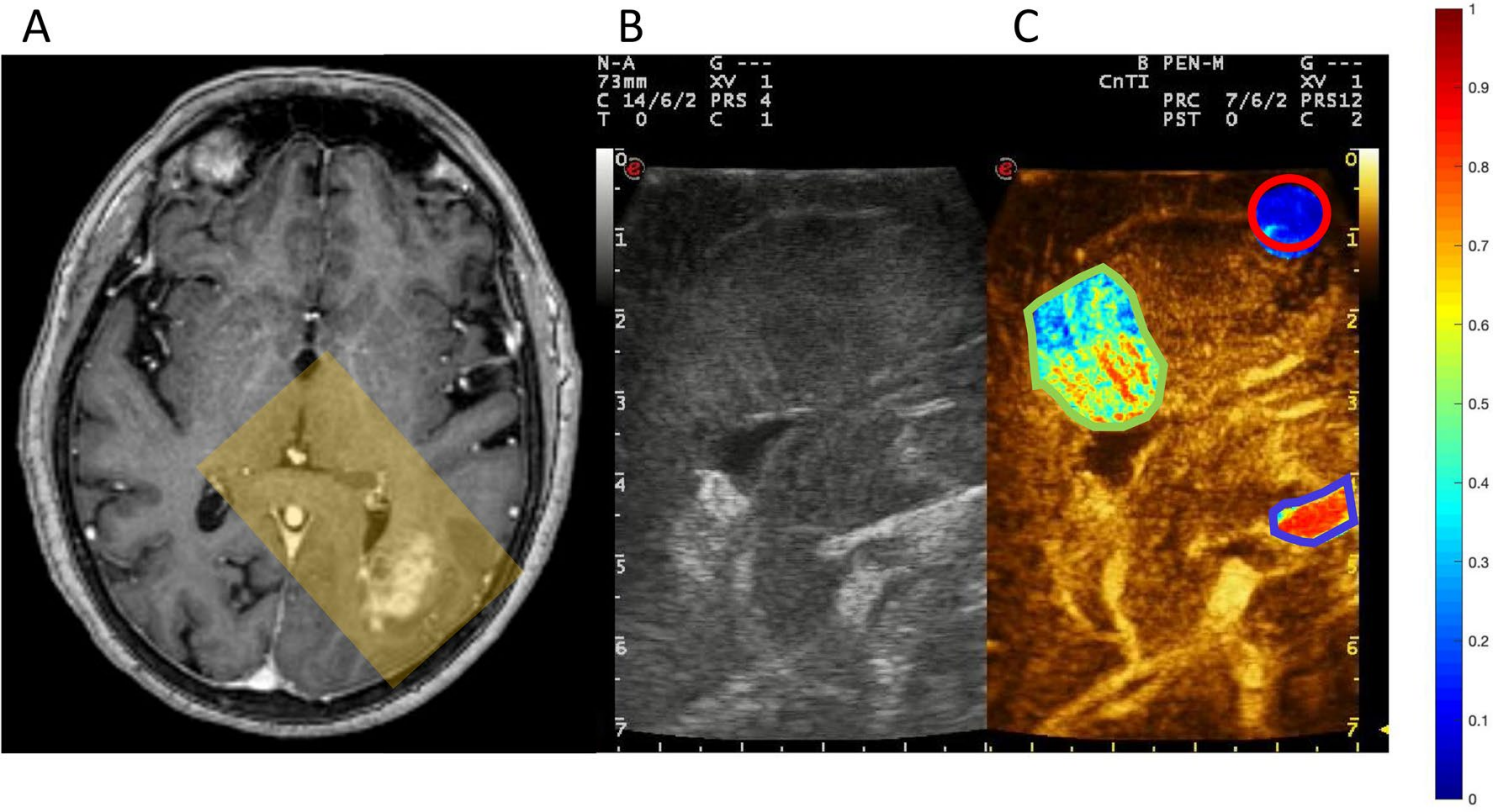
Qualitative view of microbubble density in selected regions in a case of parietal glioblastoma: (A) Gd-enhanced T1 axial MRI showing the lesion. A transparent yellow rectangle shows the plan of insonation through the craniotomy, corresponding to the US images on the right. Screenshot of the US monitor showing low mechanical index B-mode (B) and CEUS images (C). In (C) three ROIs are depicted, circled in blue (artery), green (tumor) and red (white matter), displaying MB density in JET colorimetric scale on the side.
